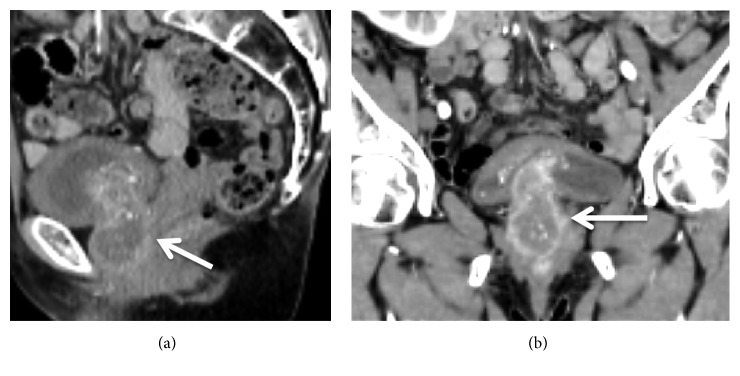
Abdominal enhanced computed tomography images showing a 3.0×5.0 cm mass with a heterogeneous contrasting effect between the urethra and bladder neck. (a) Sagittal section (arrow indicates tumor). (b) Coronal section (arrow indicates tumor).
